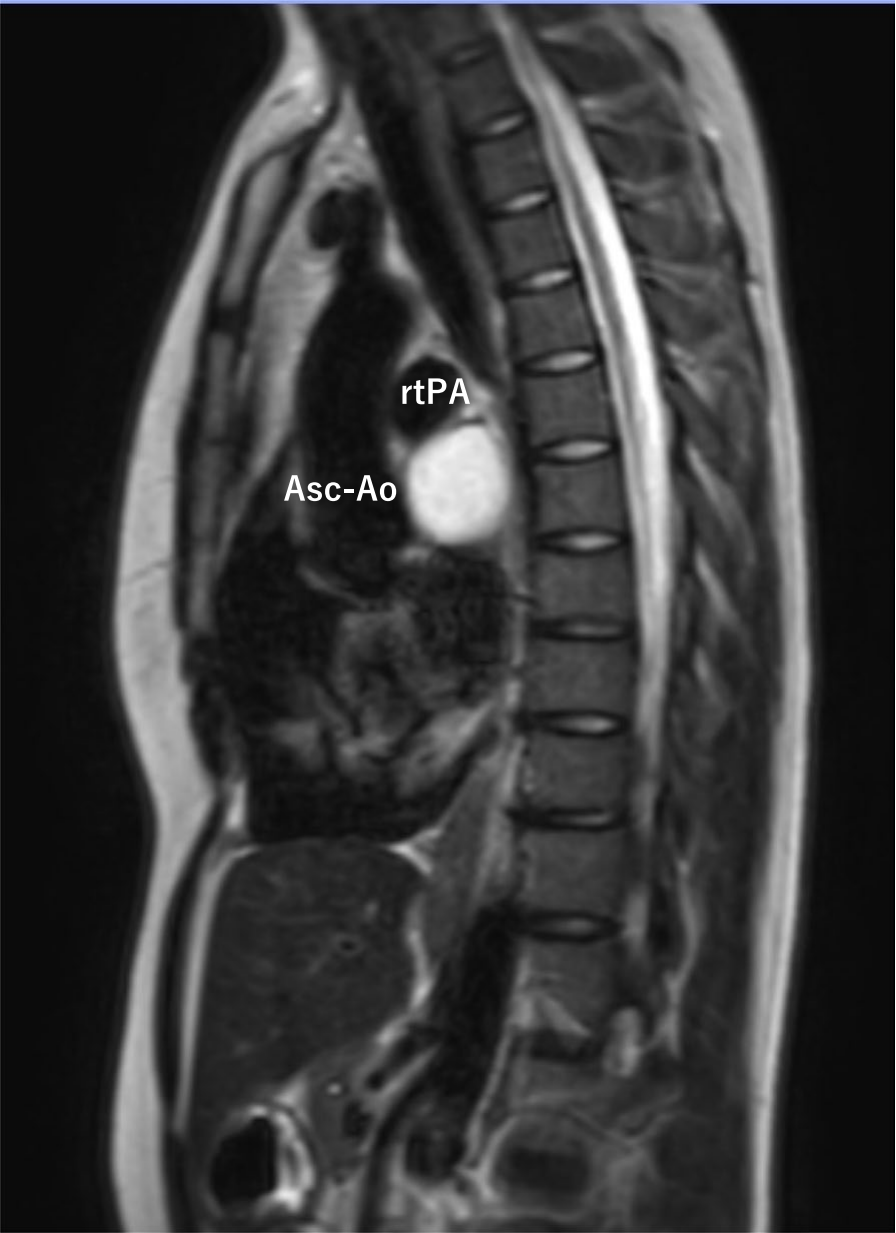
MRI indicating the location of the cyst
